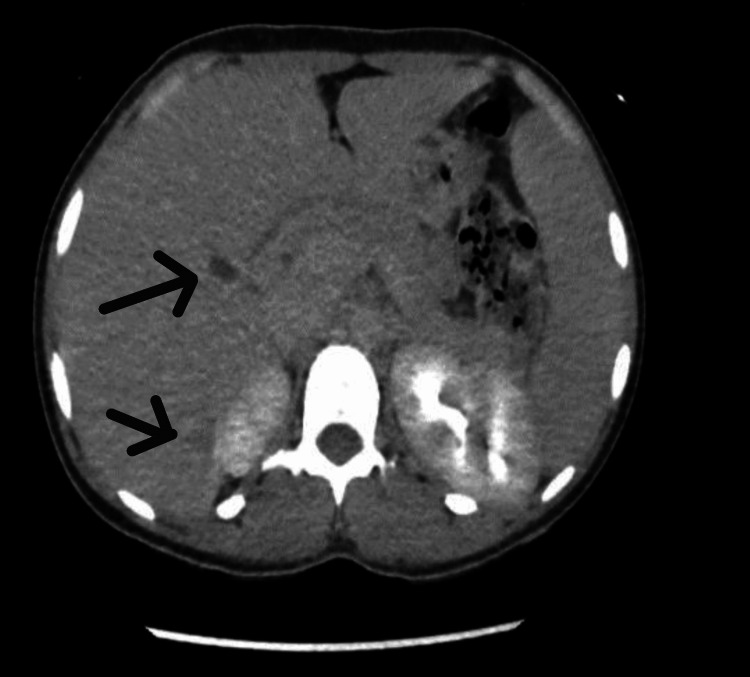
CT abdomen: hepatic microabscesses CT, computed tomography
